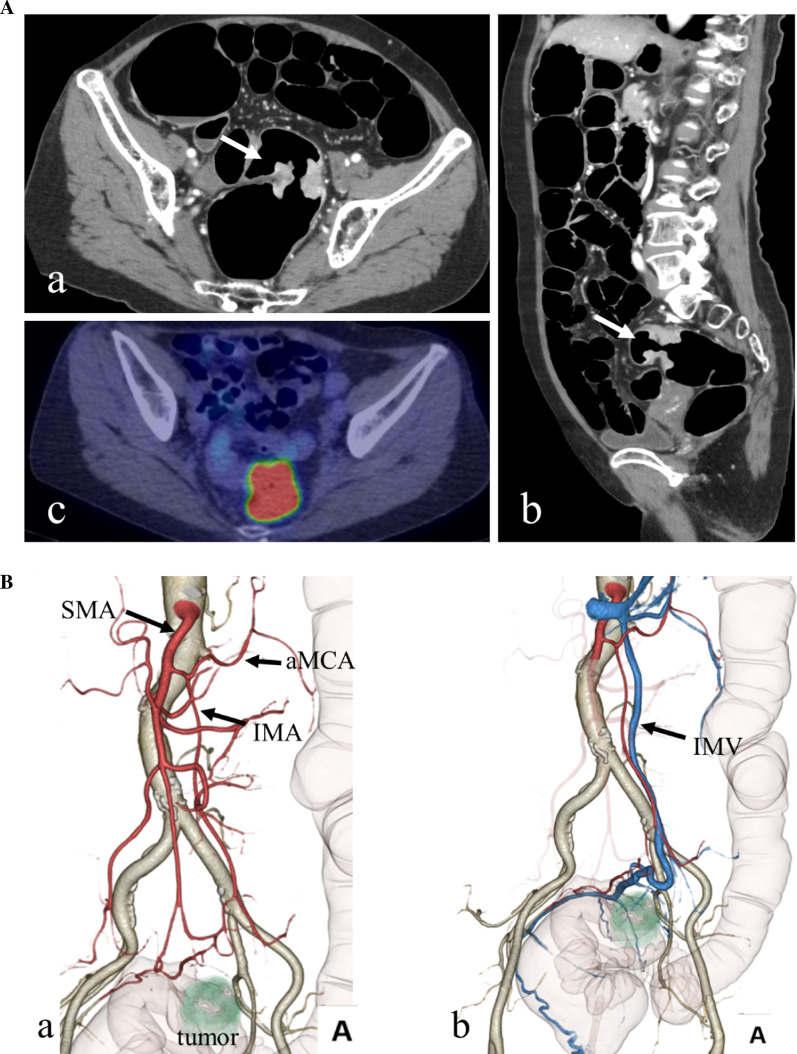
Preoperative contrast-enhanced computed tomography (CT) and PET–CT. A CT revealed wall thickening in the rectum (arrow). There were no enlarged lymph nodes or distant metastases (a: axial, b: sagittal). Positron emission tomography CT (PET–CT) revealed abnormal accumulation of FDG at the rectal tumor (c). B Three-dimensional-CT angiography reveals the IMA arising from the SMA (a). The IMA is accompanied by the IMV (b). (*IMA* inferior mesenteric artery, *SMA* superior mesenteric artery, *IMV* inferior mesenteric vein, *FDG* fluorodeoxyglucose
